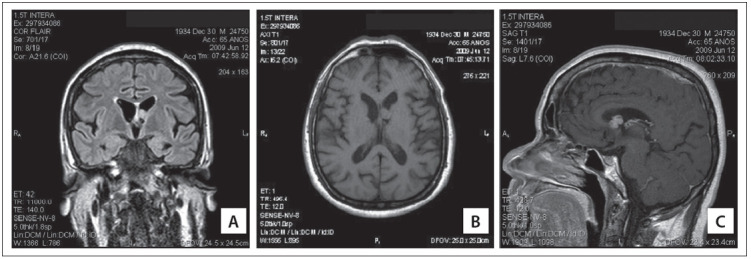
Neurological tuberous sclerosis complex features detected by means of magnetic resonance imaging (MRI) in a 1.5-T scanner. (A) Coronal and (B) axial FLAIR (fluid acquisition inversion recovery) magnetic resonance imaging (MRI), demonstrating the Monroforamen nodule, compatible with subependymal giant-cell astrocytoma, with and without administration of gadolinium contrast (GdDTPA), respectively; (C) sagittal T1 MRI after GdDTPA, showing a hyperintense signal from the tumor.
